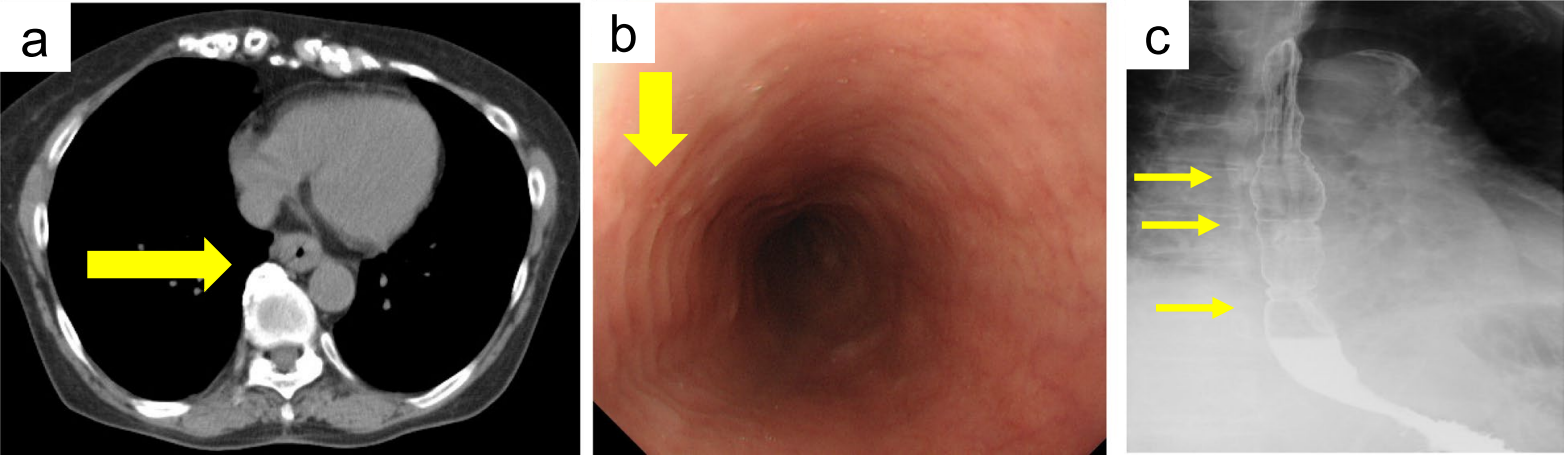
a Computed tomography scan reveals thickening of the esophageal wall extending from the upper to the middle esophagus. (arrow) (b) Upper gastrointestinal endoscopic results before benralizumab; multiple esophageal rings (trachealization) (arrow) and full circumferential mucosal edema and reduced vascular permeability are found from the upper to the middle esophagus. c Esophagography showed several ring stenoses in the middle esophagus. (arrow)
